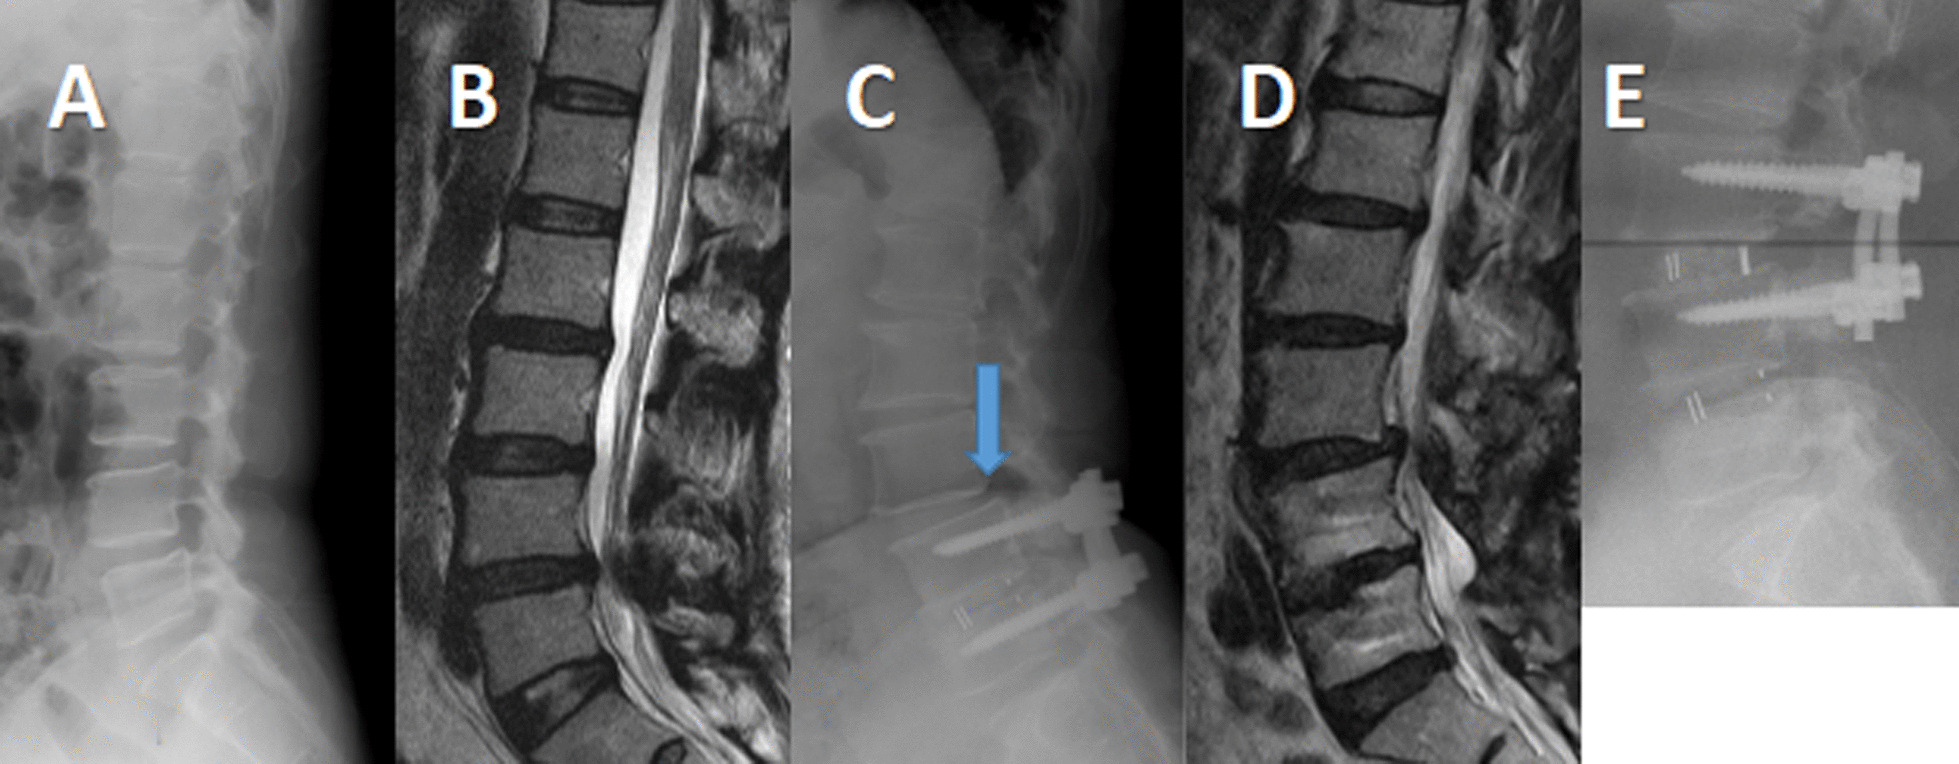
A, B Lateral X-ray and sagittal magnetic resonance image of lumbar in a 48-year-old female patient complaining of lumbar pain with right lower extremity pain. It showed L4 degenerative lumbar spondylolisthesis and L4-5 disc degeneration. C, D 47 months after TLIF, this patient again complained of low back pain with numbness in both lower extremities. Lateral X-ray and sagittal magnetic resonance image of lumbar showed L3 spondylolisthesis and L3-4, L5-S1 intervertebral disc degeneration aggravated relative to preoperative. E The patient selected TLIF revision surgery. Lateral X-ray of lumbar spine showed satisfactory L3 reduction
